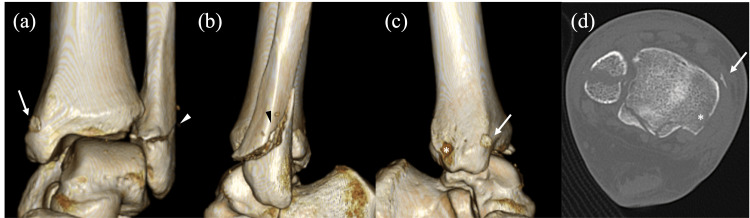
Preoperative computed tomography (CT) images of the ankle. (a-c) Three-dimensional reconstructed views. (a) Anterior view; (b) Lateral view; (c) Medial view; (d) Axial CT image. A cortical fragment consistent with the medial malleolar fleck sign (arrow) is seen anteriorly displaced from its avulsion site just proximal to the posterior colliculus (asterisk), along with a Weber B distal fibular fracture (arrowhead).
